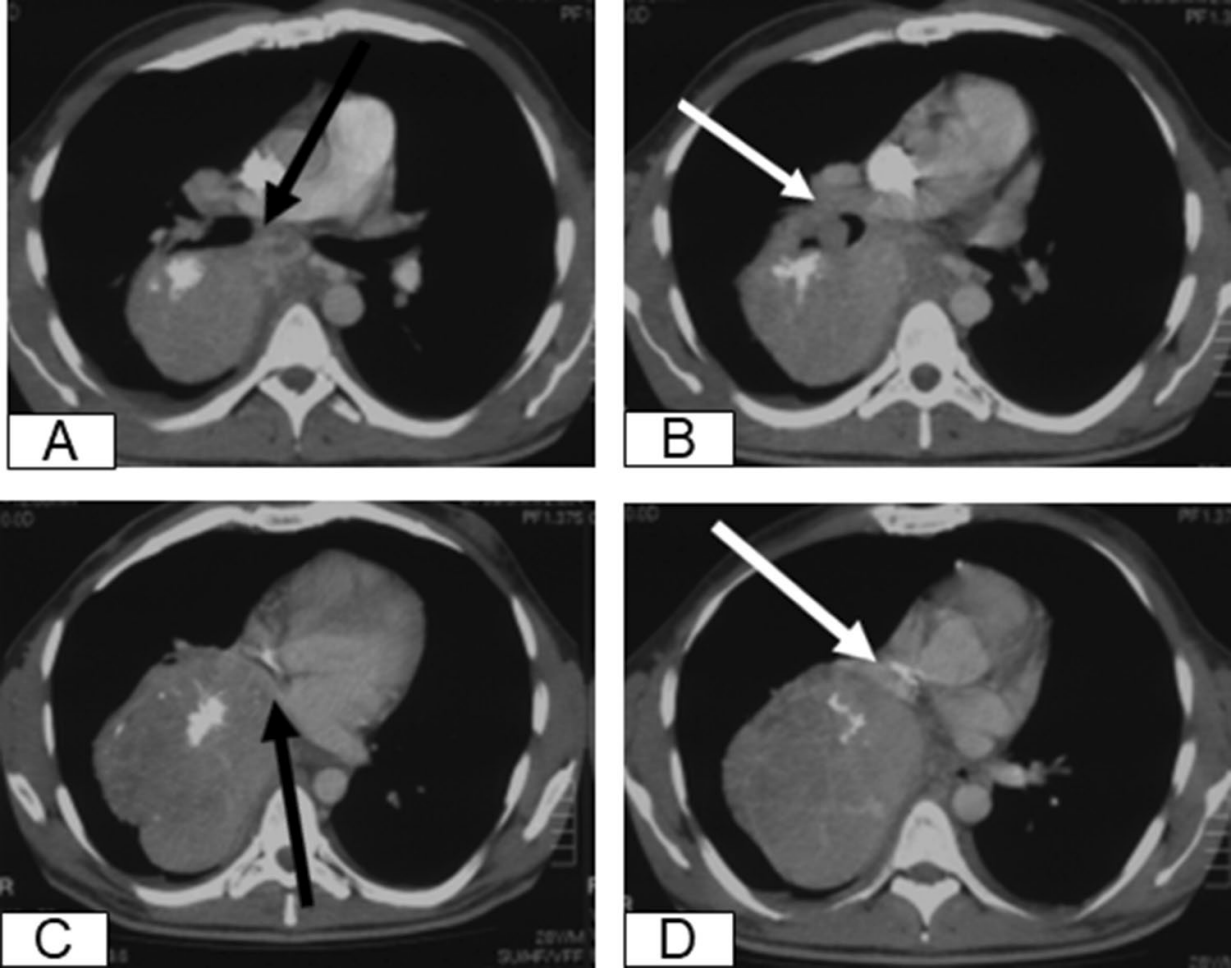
CT scan of the chest, showing close relationship to the right main bronchus (black arrow) (a), close relationship to the right main pulmonary artery (white arrow) (b), compressed upper lung vein (black arrow) (c), totally occluded right lower lung vein (white arrow) (d)
